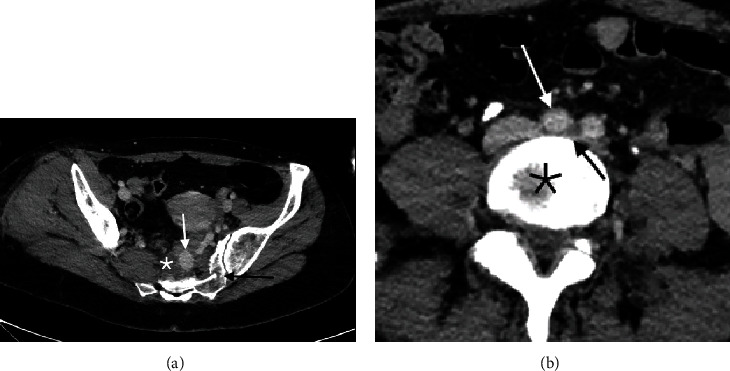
(a) Image from CT abdomen pelvis with contrast demonstrating pelvic varicosities as well as a large pseudoaneurysm (white arrow) surrounded by hematoma (star) anterior to a fractured sacrum (black arrow). (b) Additional CT image demonstrating compression of the left common iliac vein (black arrow) between the overlying right common iliac artery (white arrow) and the underlying vertebral body (star), typical of MTS anatomy.
